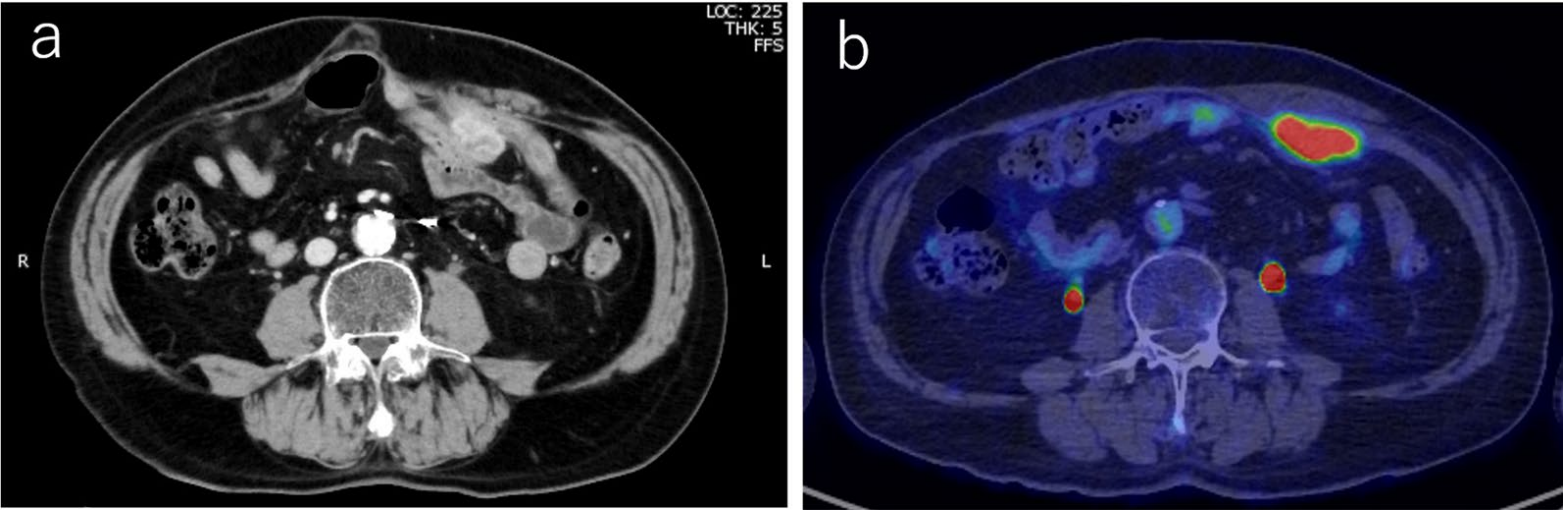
Radiological features of the case. a Abdominal computed tomography (CT) identified a mass near the stoma just below the abdominal wall. b There was no other nodule suggestive of peritoneal dissemination. The abdominal mass was removed along with the stoma, abdominal wall, mesh, and the associated portion of the small intestine
